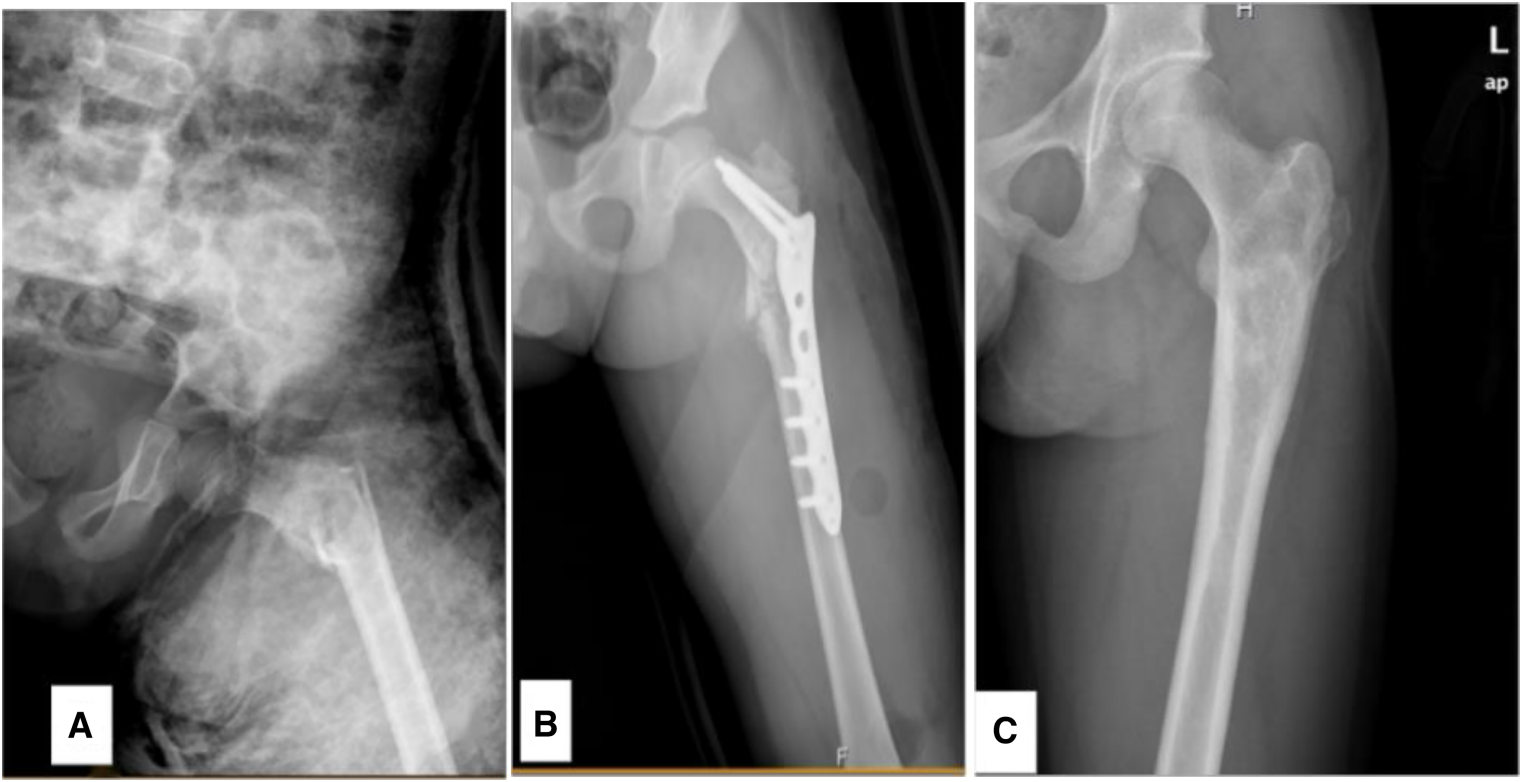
An 8.9-year-old male diagnosed with a SBC in the proximal left femur concomitant with a fracture. (A) Preoperative radiograph. (B) Radiograph taken 3 days following biopsy, curettage, LCP-PHP procedure, and bone grafting. (C) Radiograph captured 6.2 years post-surgery.
